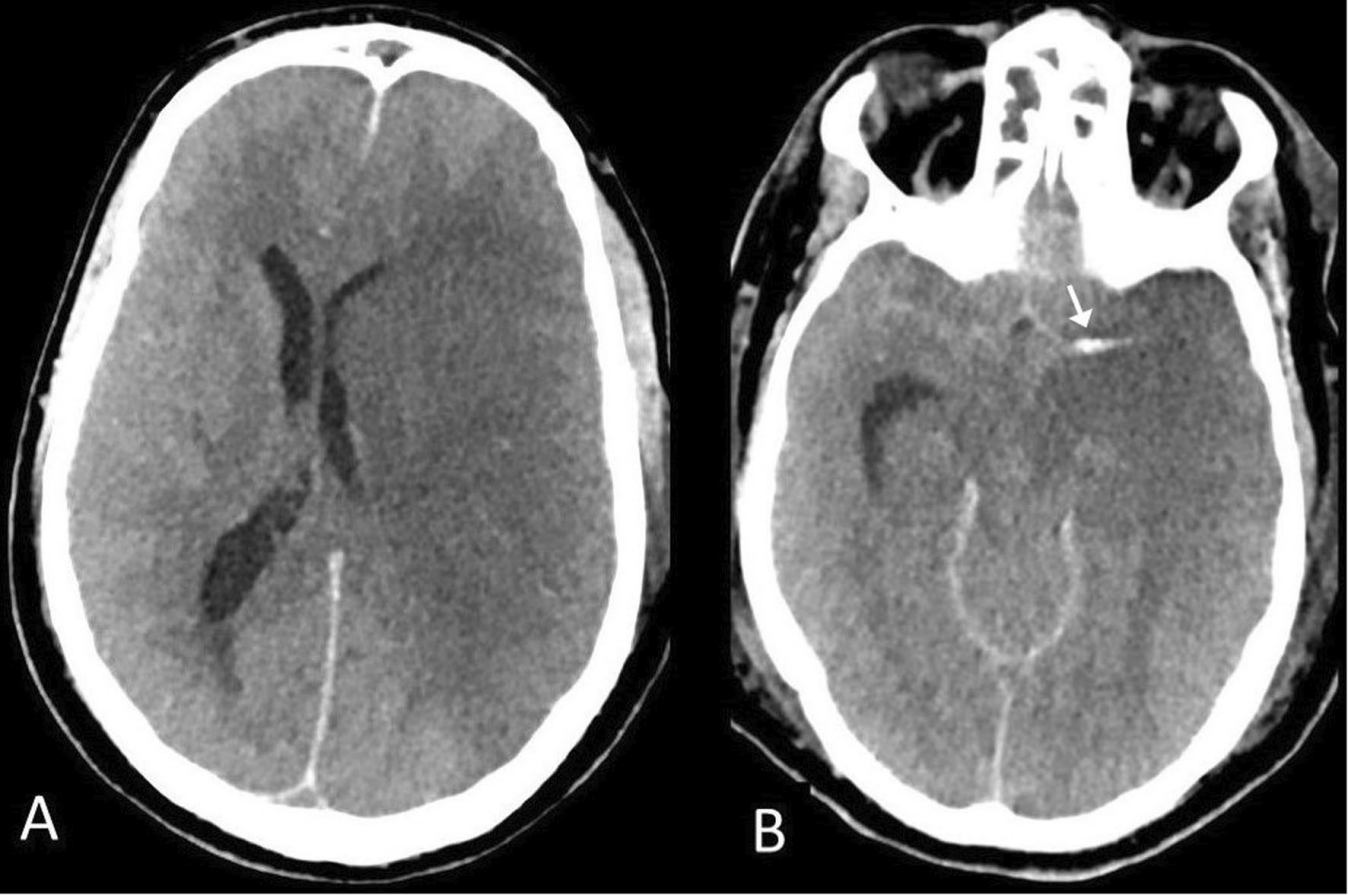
Acute ischemic infarct. 49-year-old man with COVID-19 transferred to ICU for mechanical ventilation, his neurologic condition suddenly deteriorated and the patient was found with bilaterally fixed and dilated pupils. a Axial CT image demonstrating large left MCA infarction. b Hyperdense left MCA is seen the proximal M1 segment of the left MCA (arrow), suggesting thrombus
